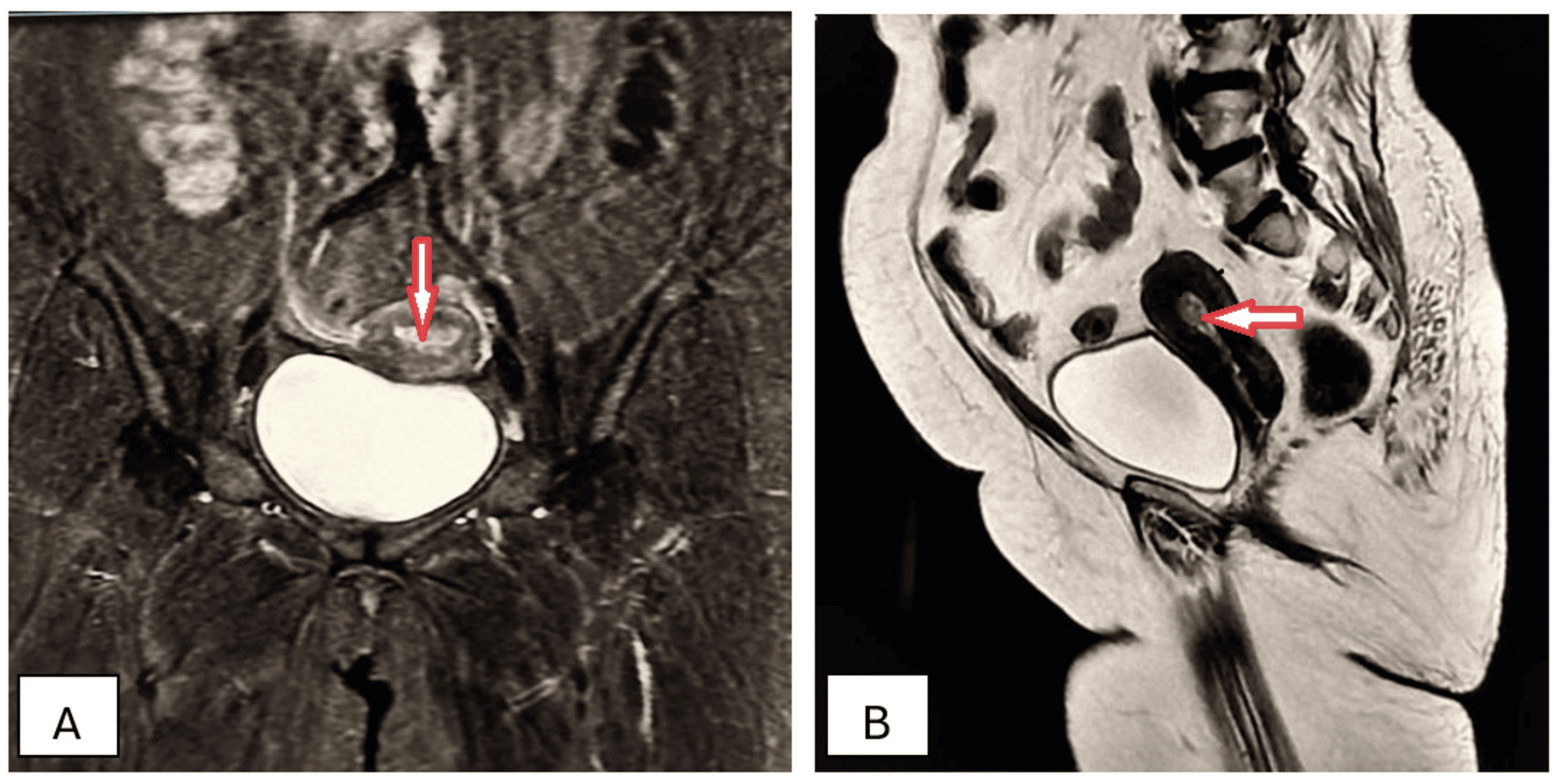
Sessile polyp of endometrium (arrows). Coronal STIR (A) and sagittal (B) T2W MRI imaging show a well-defined isointense mass in the endometrial cavity attached to the anterior wall, consistent with a sessile endometrial polyp. T2W: T2-weighted; STIR: short tau inversion recovery
